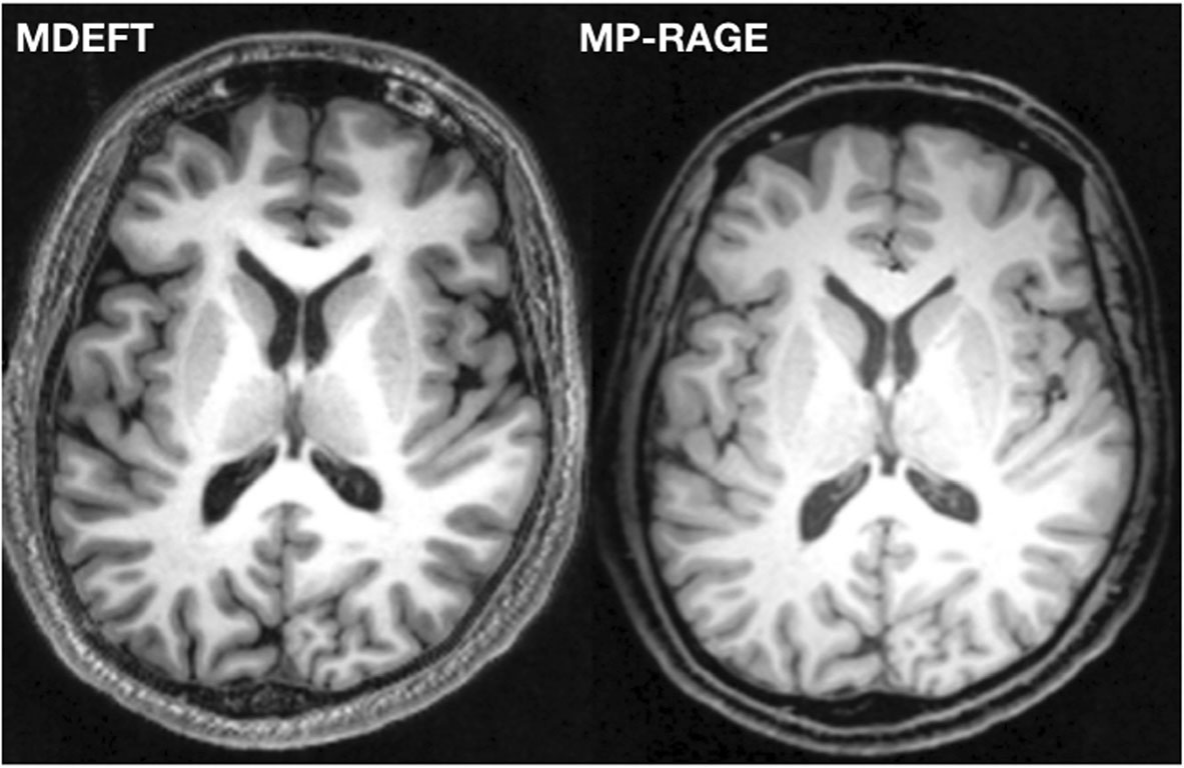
Two different MR pulse sequences were applied to acquire high-resolution 3D T1-weighted images: MP-RAGE and MDEFT pulse sequence
